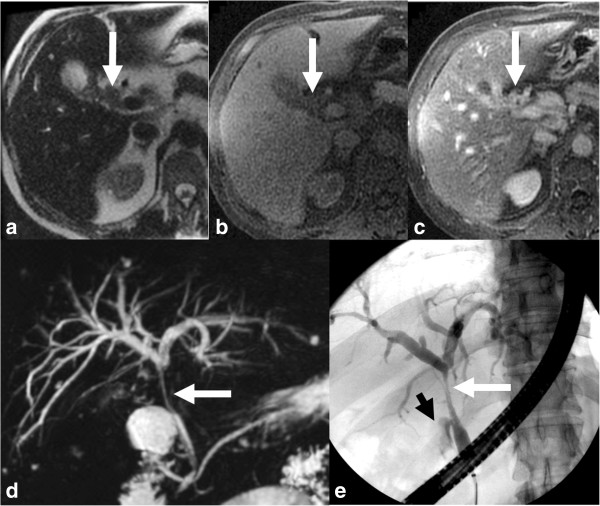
Perihilar CCA arising from the cystic duct and proximal gall bladder (arrows) with invasion of common hepatic duct. The pCCA is mildly hyperintense on T2-weighted image (a), hypointense on T1-weighted image (b) and shows post contrast enhancement (c). Both MRCP (d) and ERCP (e) demonstrate the stricture of the common hepatic duct till the confluence above and cystic duct insertion below. The gall bladder is not visualized on ERCP and the irregularity of the proximal cystic duct (arrowhead) is due to the tumor.
